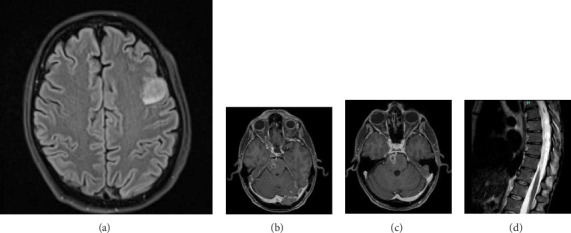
(a) Preoperative magnetic resonance imaging showing a hyperintense lesion in the left frontal opercular region on FLAIR sequences. (b and c) In contrast-enhanced sequences, a right paramedian pontine lesion involving the trigeminal nerve and Meckel's cave was evident. (d) The spine MRI has also revealed a signal of spinal cord hyperintensity from T3 to the conus.
